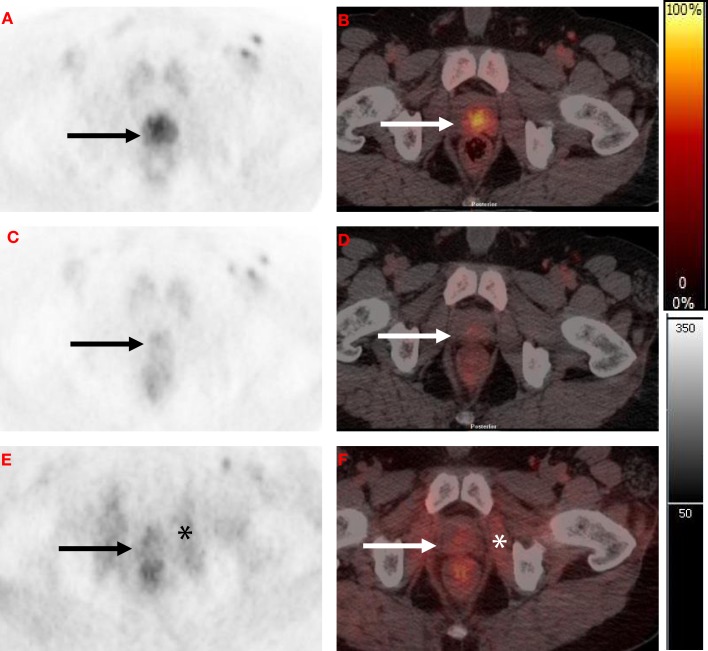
Axial [^11^C]choline PET and fused PET/CT at level of the prostate. (A,B) Baseline scan with focal activity in the peripheral zone (black and white arrows). (C,D) Post-neoadjuvant androgen deprivation therapy (NAD) scan (8–10 weeks after initiating NAD) with a marked reduction in [^11^C]choline uptake in the peripheral zone. (E,F) Post- radiotherapy combined with concurrent androgen deprivation therapy (RT-CAD) scan (4 months after completion of RT-CAD) with a further reduction in prostate activity and increased obturator internus muscular activity (black and white asterisk).
